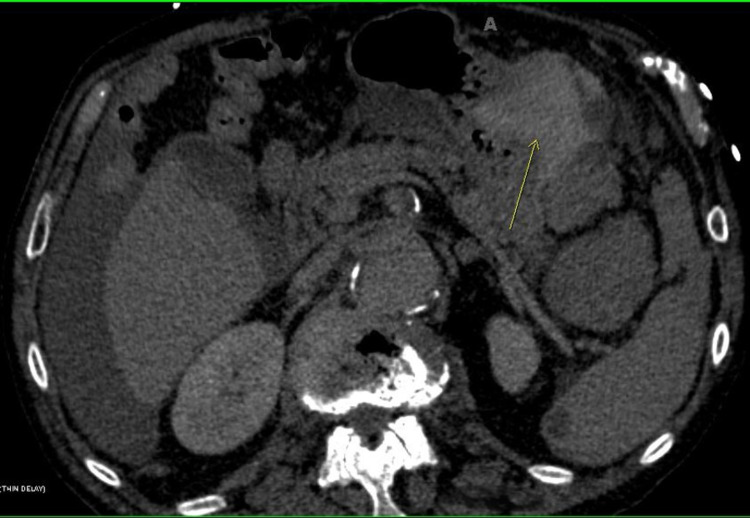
Computed tomography (CT) of the abdomen and pelvis revealed a ruptured splenic capsule (arrow).
